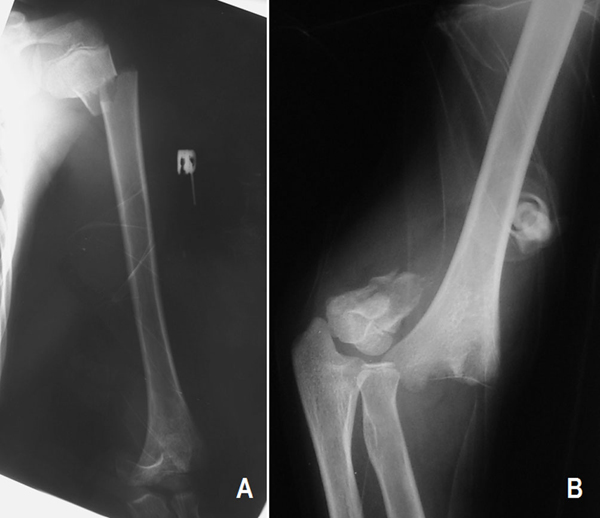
Preoperative (A) anteroposterior roentgenogram of the left upper extremity and (B) lateral roentgenogram of the left elbow.
